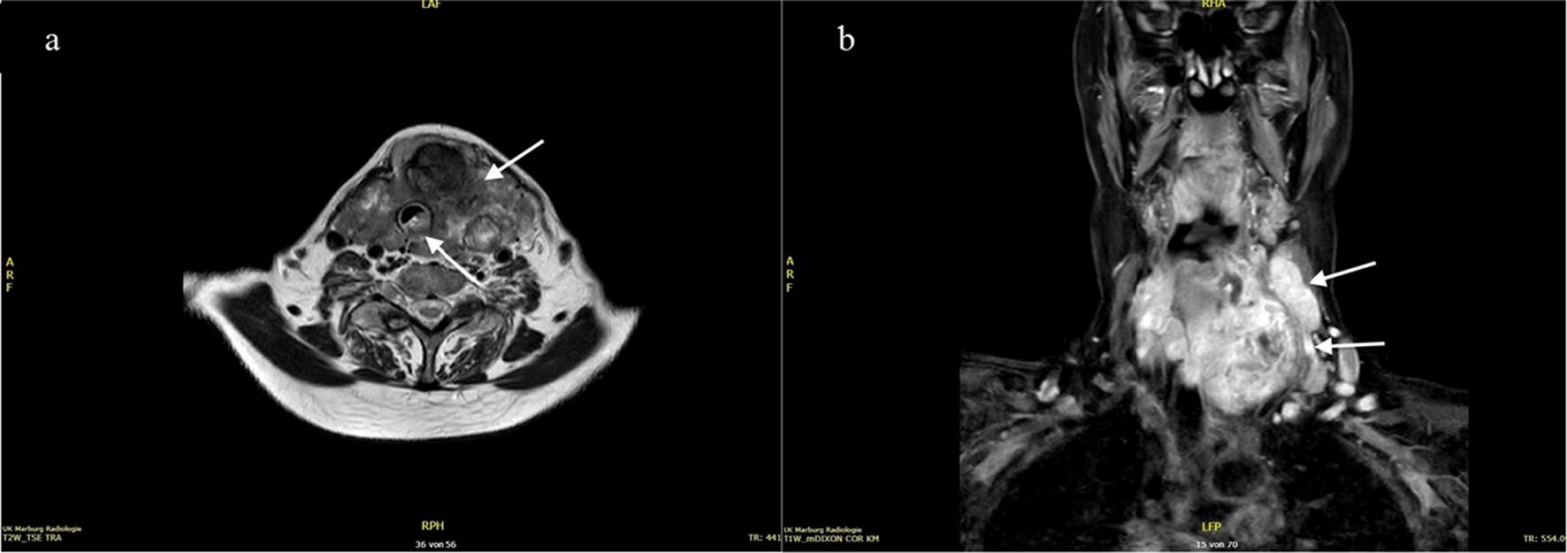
Patient 1# ATC of the left thyroid lobe: MRI at diagnosis. a Left-sided thyroid tumor with tracheal infiltration. b Carotid artery walled by tumor and lymph node metastases
